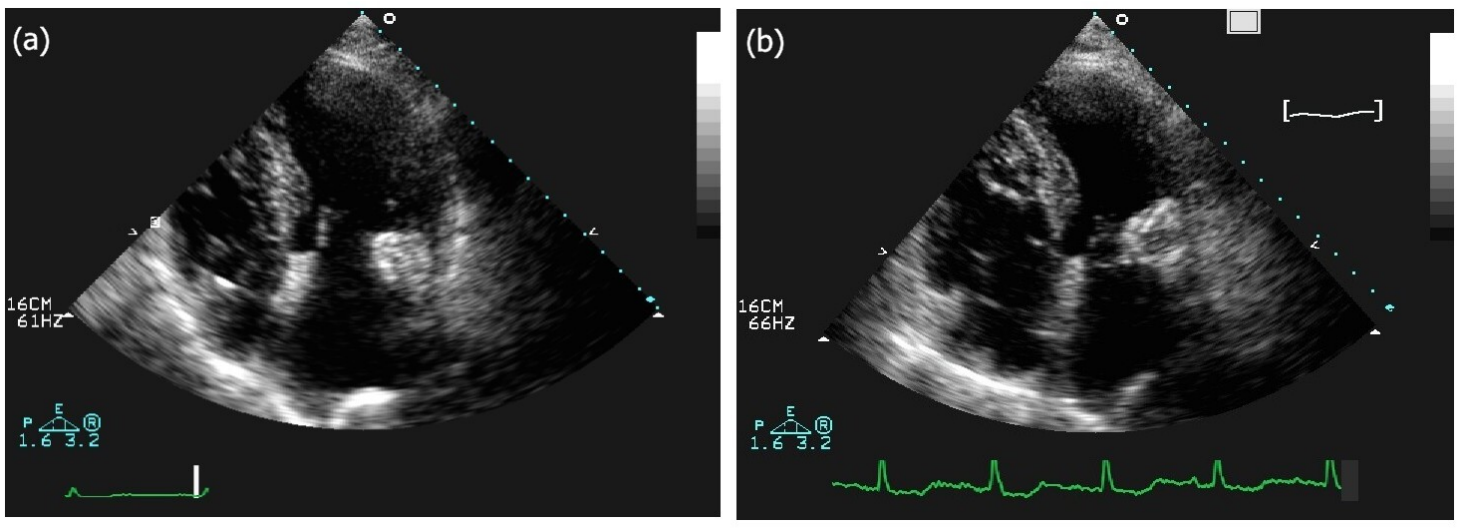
Two-dimensional echocardiogram, apical four-chamber view. (a) Pre-operative: a round echodense large mass attached to the calcified mitral annulus is seen. (b) Postoperative: a smaller round echodense mass attached to the calcified annulus is seen.
